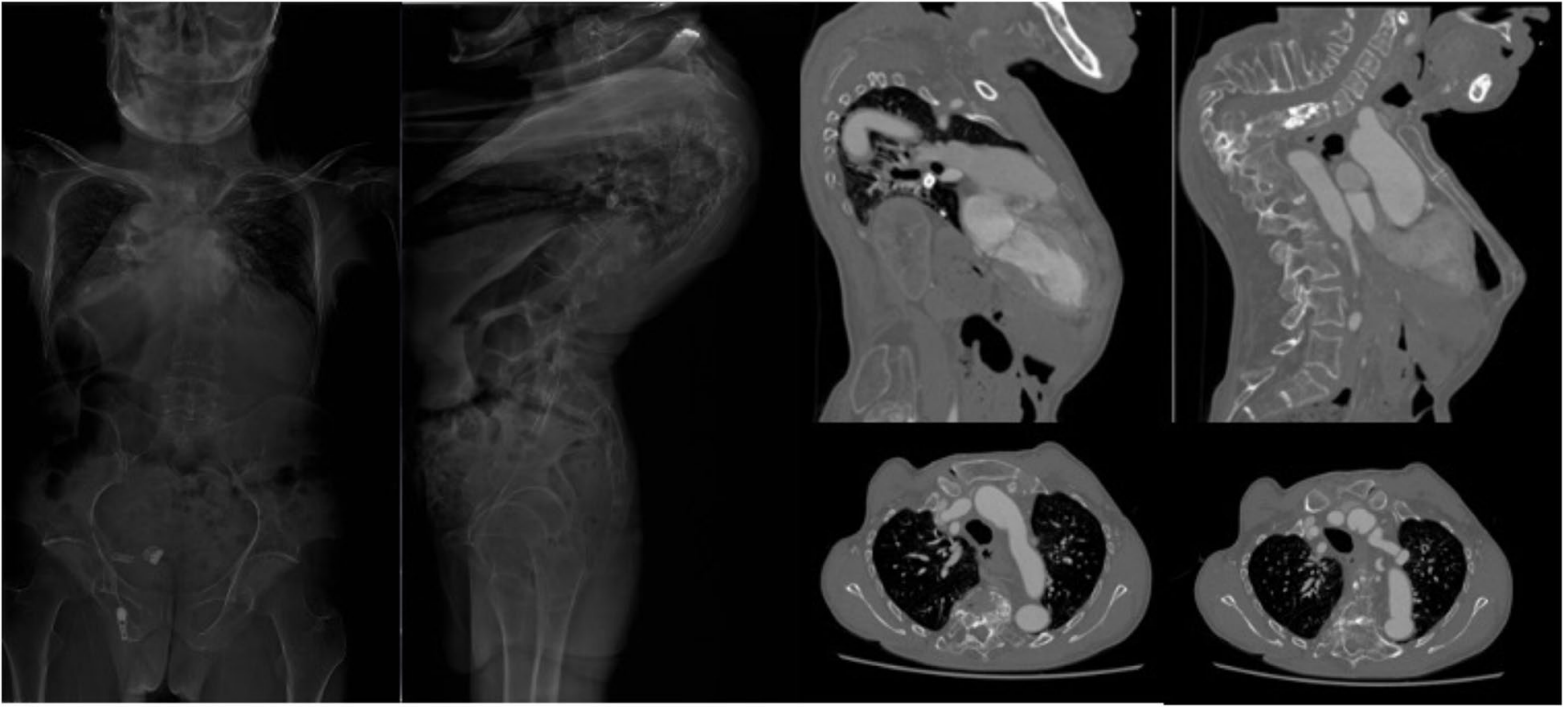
A 55 years old patient presented with lower limb weakness. Anterior column collapse in upper thoracic region. The kyphotic angle of T4-6 was 73.1° and SVA was measured 1.7 cm. CT scans showed that the aorta was located at the antero-lateral position of the first third of the apex vertebra (Grade 1)
